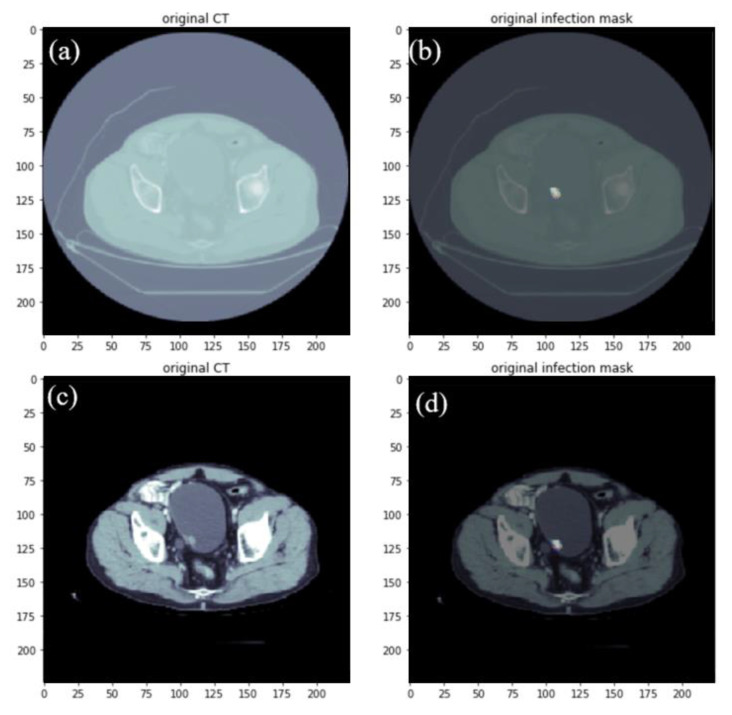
Before adjusting the HU value of image data, (a) the original CT of the hospital (b) mark the focus (white color light is the location of the focus). After adjusting the HU value of the image data (c), the original CT of the hospital (d) labeled the lesion (white color light is the location of the lesion).
